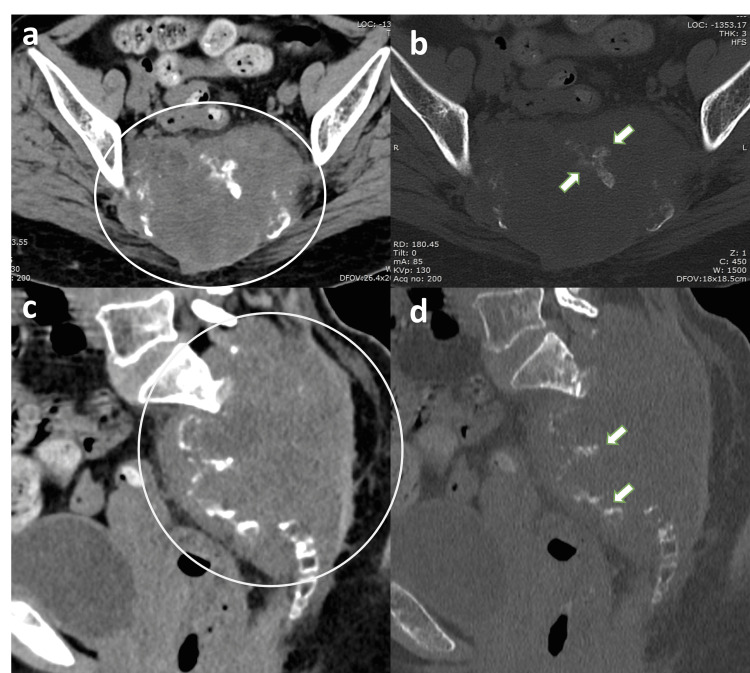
CT demonstration of sacral destruction and internal calcifications Axial and sagittal reformatted CT images in soft tissue (a, c) and bone windows (b, d) demonstrate a lobulated destructive lytic lesion centered within the sacrum (circled area). Extensive cortical destruction and expansile remodeling are present, with multiple internal calcific foci (arrows) representing matrix mineralization.
